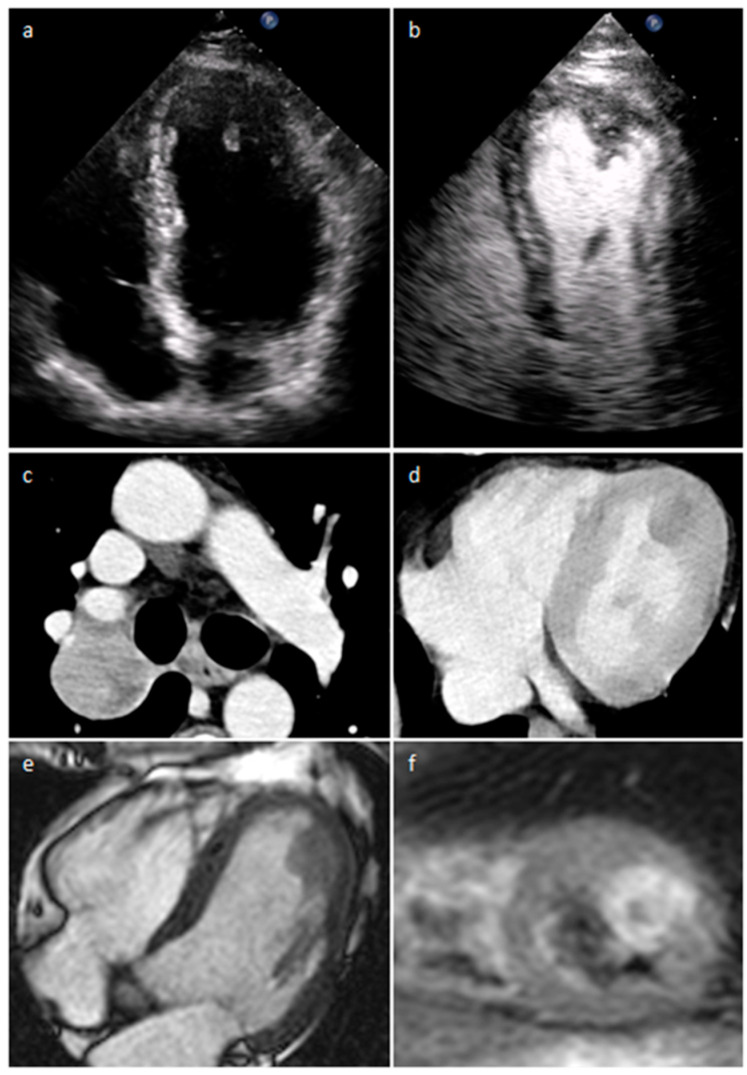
Left ventricle metastasis on precontrast (a) and postcontrast (b) transthoracic echocardiogram images; primitive lung lesion (c) and cardiac metastasis on venous phase CT (d); cardiac secondary tumor on four-chamber cine (e) and short-axis T2 STIR (f) MR imaging.
